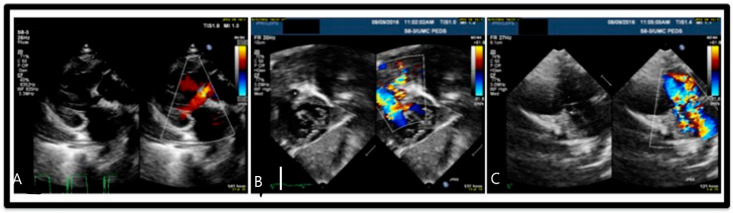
Echocardiogram: (A) Long axis view of ventricular septal defect (VSD) demonstrating pulmonary vessel stenosis. (B) Subcostal four chamber view of VSD with aorta overriding pulmonary artery and feeding into right ventricle. (C) View of double outlet pulmonary artery and aorta from right ventricle with VSD present.
